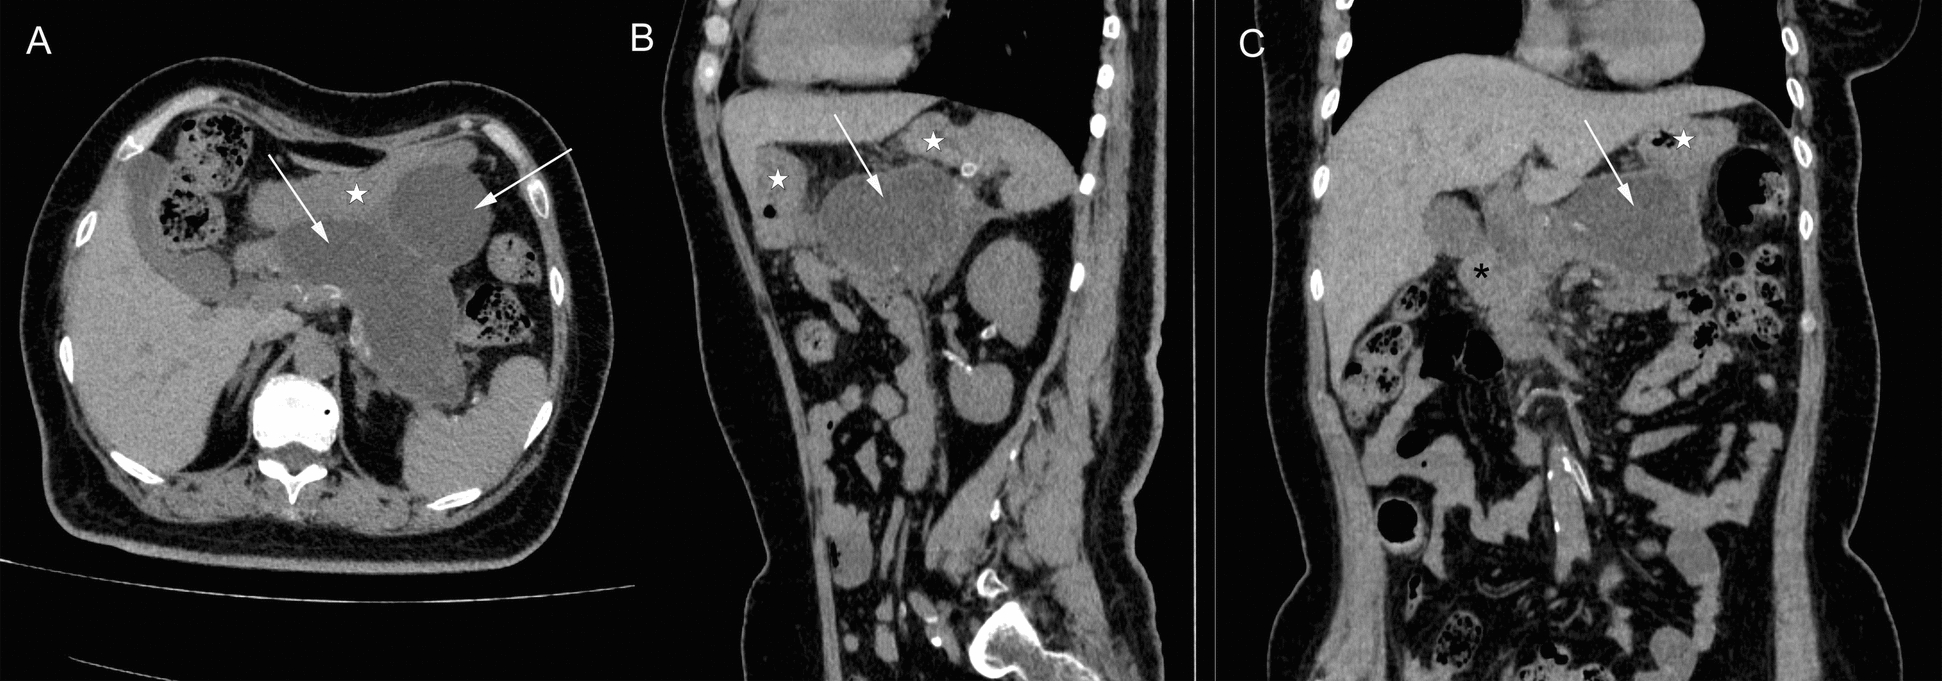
Abdominal computed tomography scan on admission day. Axial (A), sagittal (B), and coronal (C) computed tomography showing a heterogeneous, fully encapsulated necrosis collection in the peripancreatic area (arrows); stars, stomach; asterisk, duodenum
